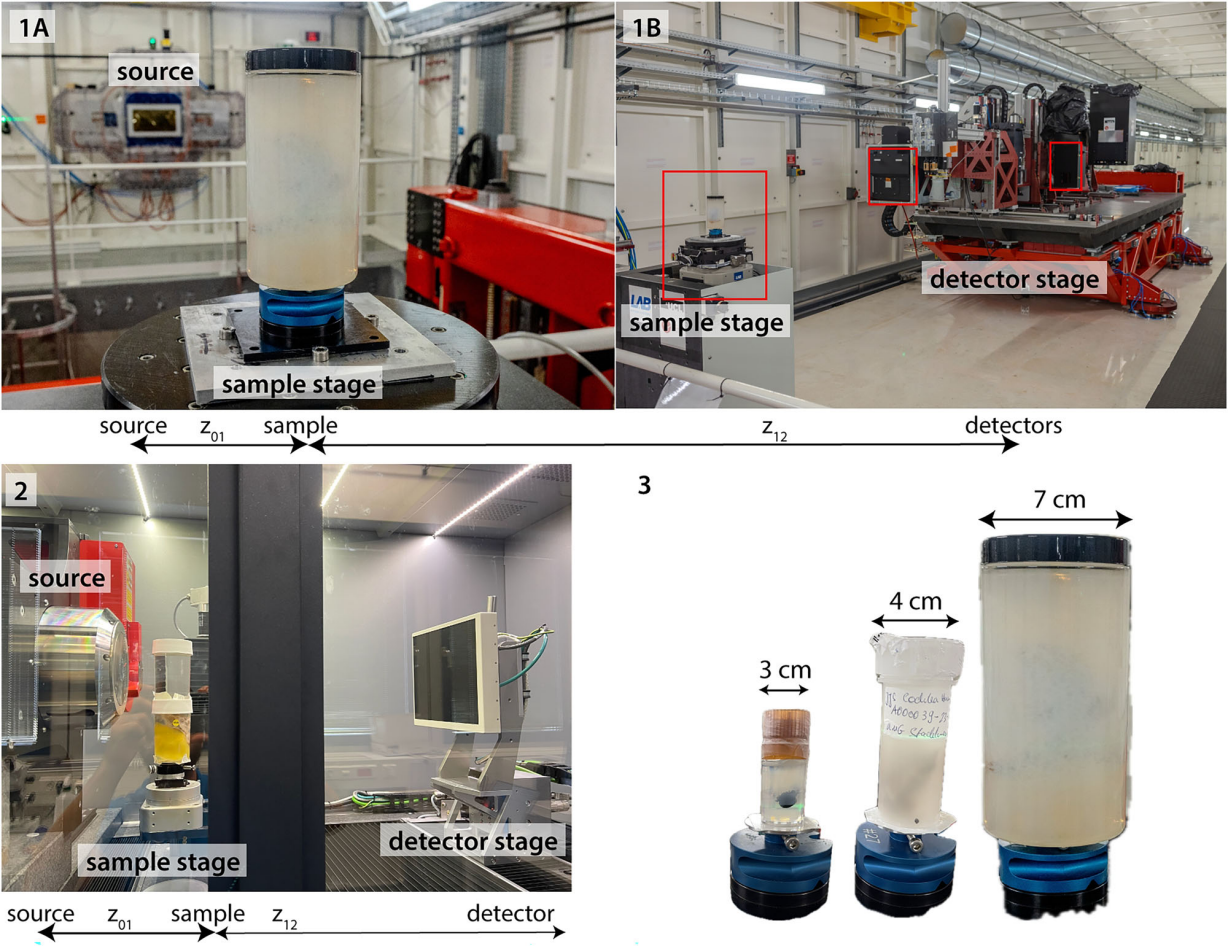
Synchrotron and *μ*-CT setups. (1) BM18 beamline, ESRF, showing (A) exit window and sample stage and (B) sample stage and detector stage with different detector-lens combinations. The flatfield images are usually taken in a sample-free region of the sample jar. (2) μ-CT setup with source, sample stage and detector. The flatfield images are taken in a control jar that is filled with the same solvent as the sample jar. (3) Sample jars used for different sample sizes such as the excised cochlea and the temporal bone.
